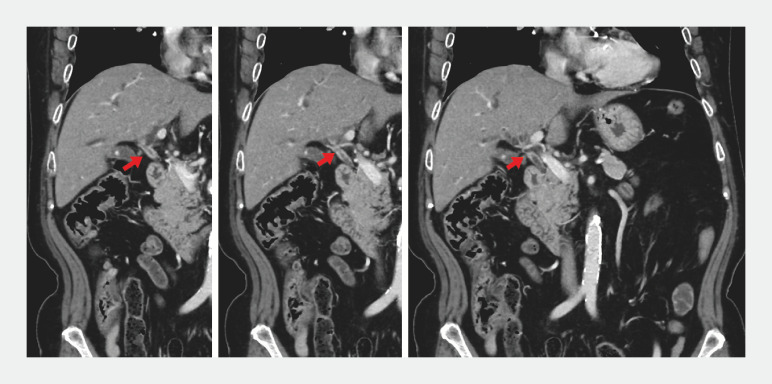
Contrast-enhanced computed tomography images showing a stricture and wall thickening
